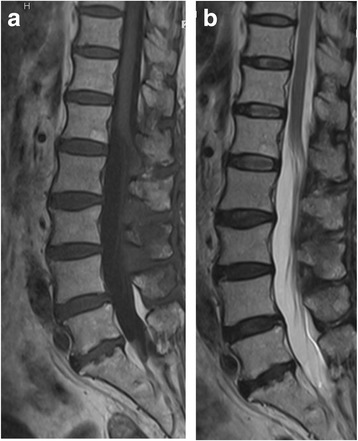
Sagittal view of T1-weighted (a) and T2-weighted (b) MRI image and a T2- weighted image at the L3/L4 level 47 days after surgery. Note that the abscess has disappeared
